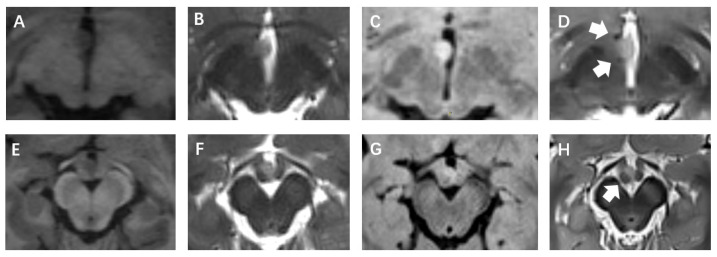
(A,E) T1-MPRAGE. (B,F) 3D-T2WI TSE. (C,G) 3D-T2WI FLAIR. (D,H) T2-STIR. The white arrows in (D) point to the fornix and mammillothalamic tract, respectively. In (H), it points to the mammillary body.
